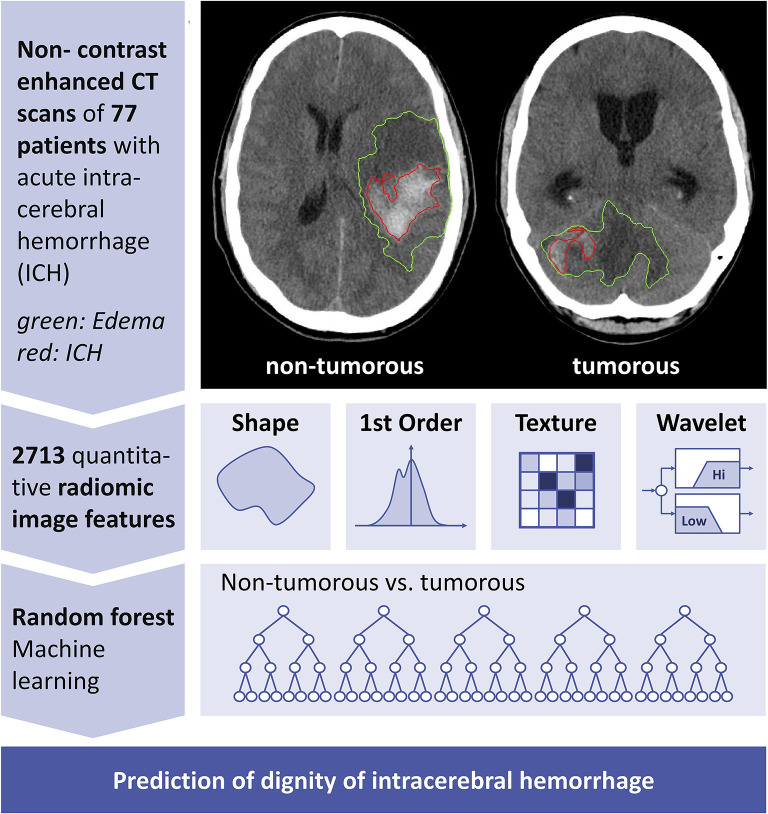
Conceptual overview of proposed neoplastic intracerebral hemorrhage prediction. Conceptual overview of the proposed machine learning approach showing the major processing steps: CT-based image acquisition and segmentation, feature extraction (*n* = 2,713), and statistical learning (random forest algorithm). NECT, non-contrast-enhanced computed tomography; ICH, intracerebral hemorrhage; PHE, perihematomal edema.
